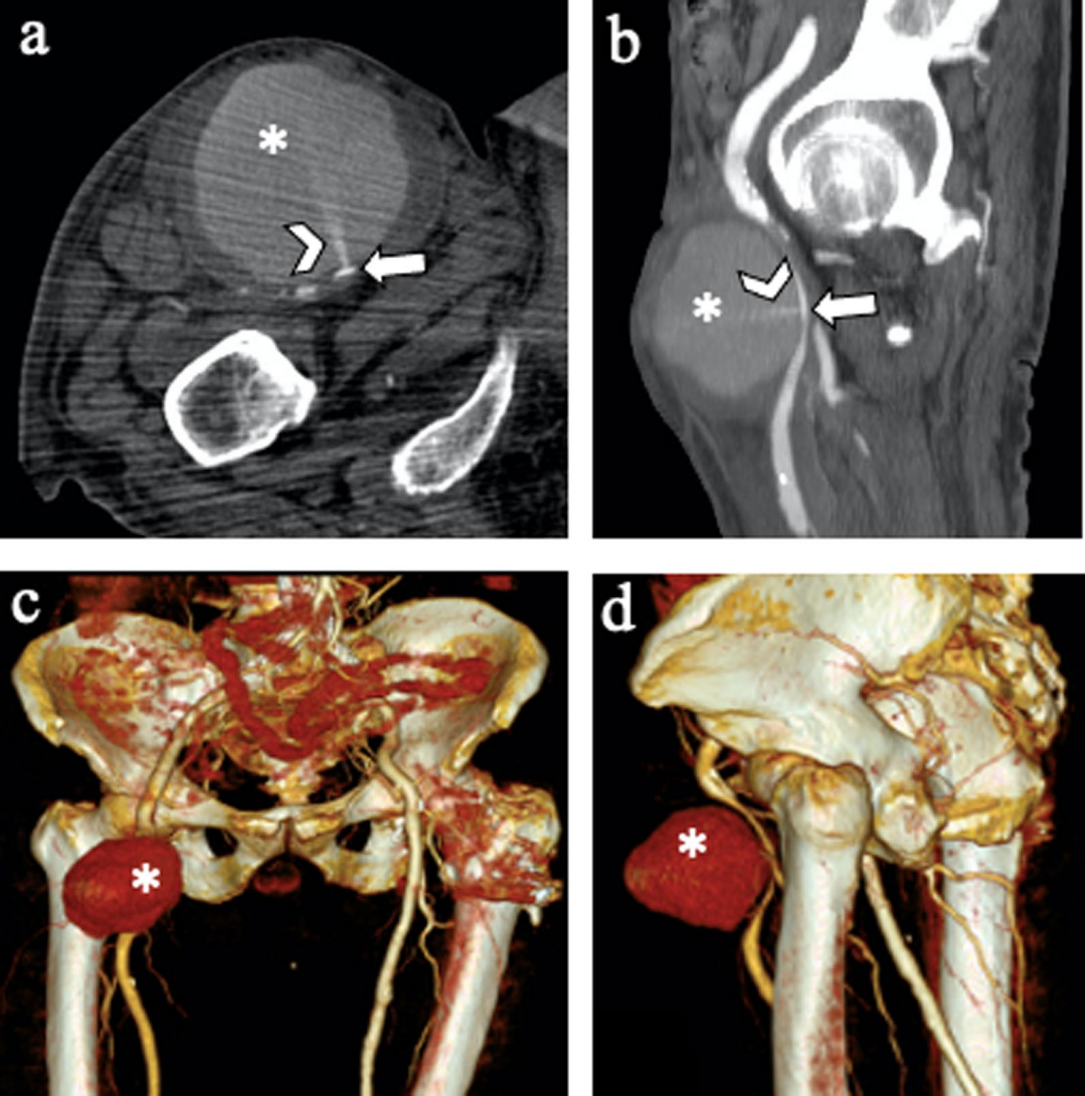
75-year-old man who underwent EVAR with right superficial femoral artery access. a Axial, (b) sagittal, and (c, d) volume rendering reconstruction CT angiography images show an access site complication: a pseudoaneurysm (*) of the right superficial femoral artery (arrows). It is also possible to see a contrast jet originating from the artery (arrowheads)
